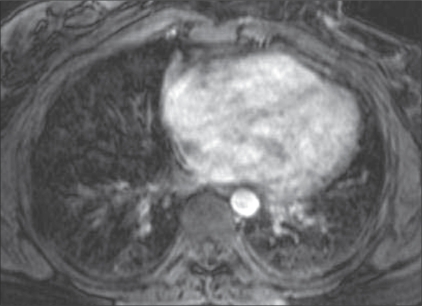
Posttreatment, postcontrast axial T1W MRI image after treatment reveal no abnormal enhancement of the myopericardium
